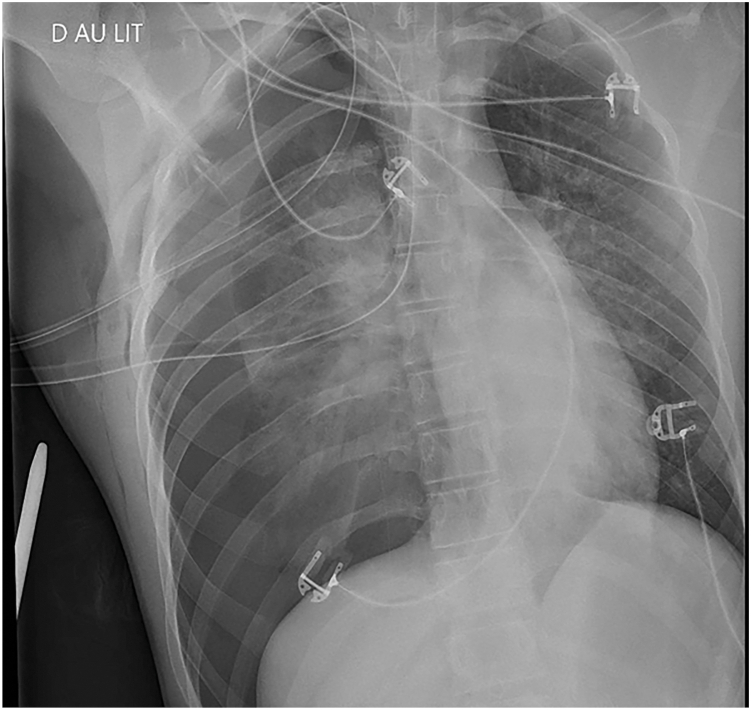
Initial chest radiograph showing persistent right pneumothorax despite placement of two chest drains.
